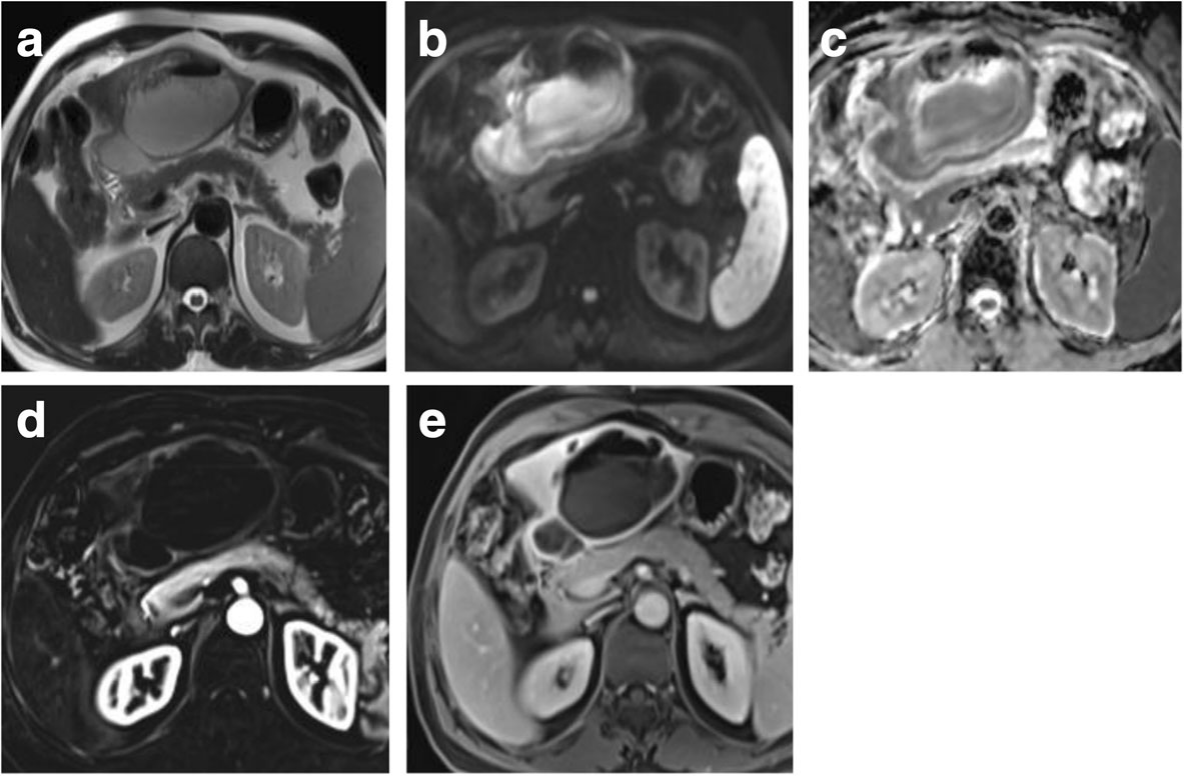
Hepatic focal lesion after TACE. a T2-WI shows a well-defined cystic lesion at the treated focal lesion with air/fluid level. b, c DWI and ADC show central areas of true restriction. d Subtracted T1-WI arterial phase shows peripheral smooth wall enhancement of the lesion. e Post-contrast T1-WI delayed phase shows peripheral smooth wall enhancement of the lesion. Overall post-treatment assessment is LR-TR nonviable with post-therapy abscess formation
